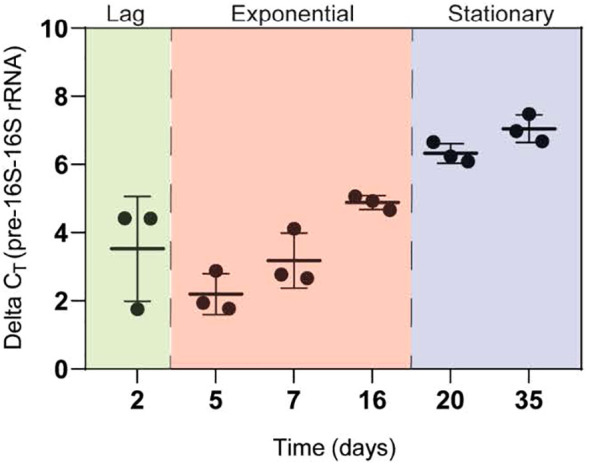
▵CT measured at different stages of in vitro growth. The progression from active replication in the logarithmic phase to the metabolically less active stationary phase is demonstrated by increasing delta CT values thorughout growth. Determination of the putative phases of replication is informed by the literature and the growth curve previously established using Mtb 16S rRNA.
